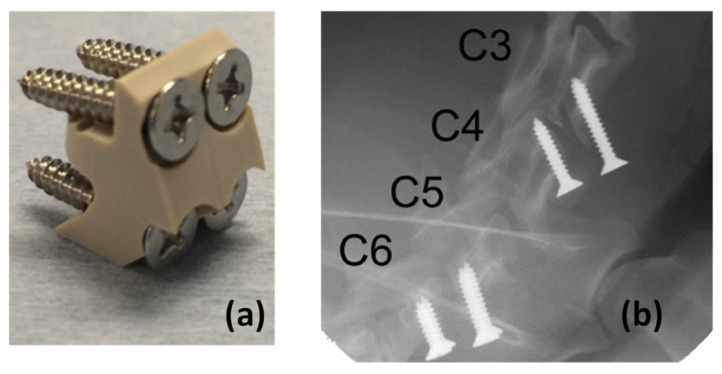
(a) Interbody spacer device and (b) postsurgical X-ray examination. Reproduced with permission from [48]. Copyright 2017, PLoS ONE.
